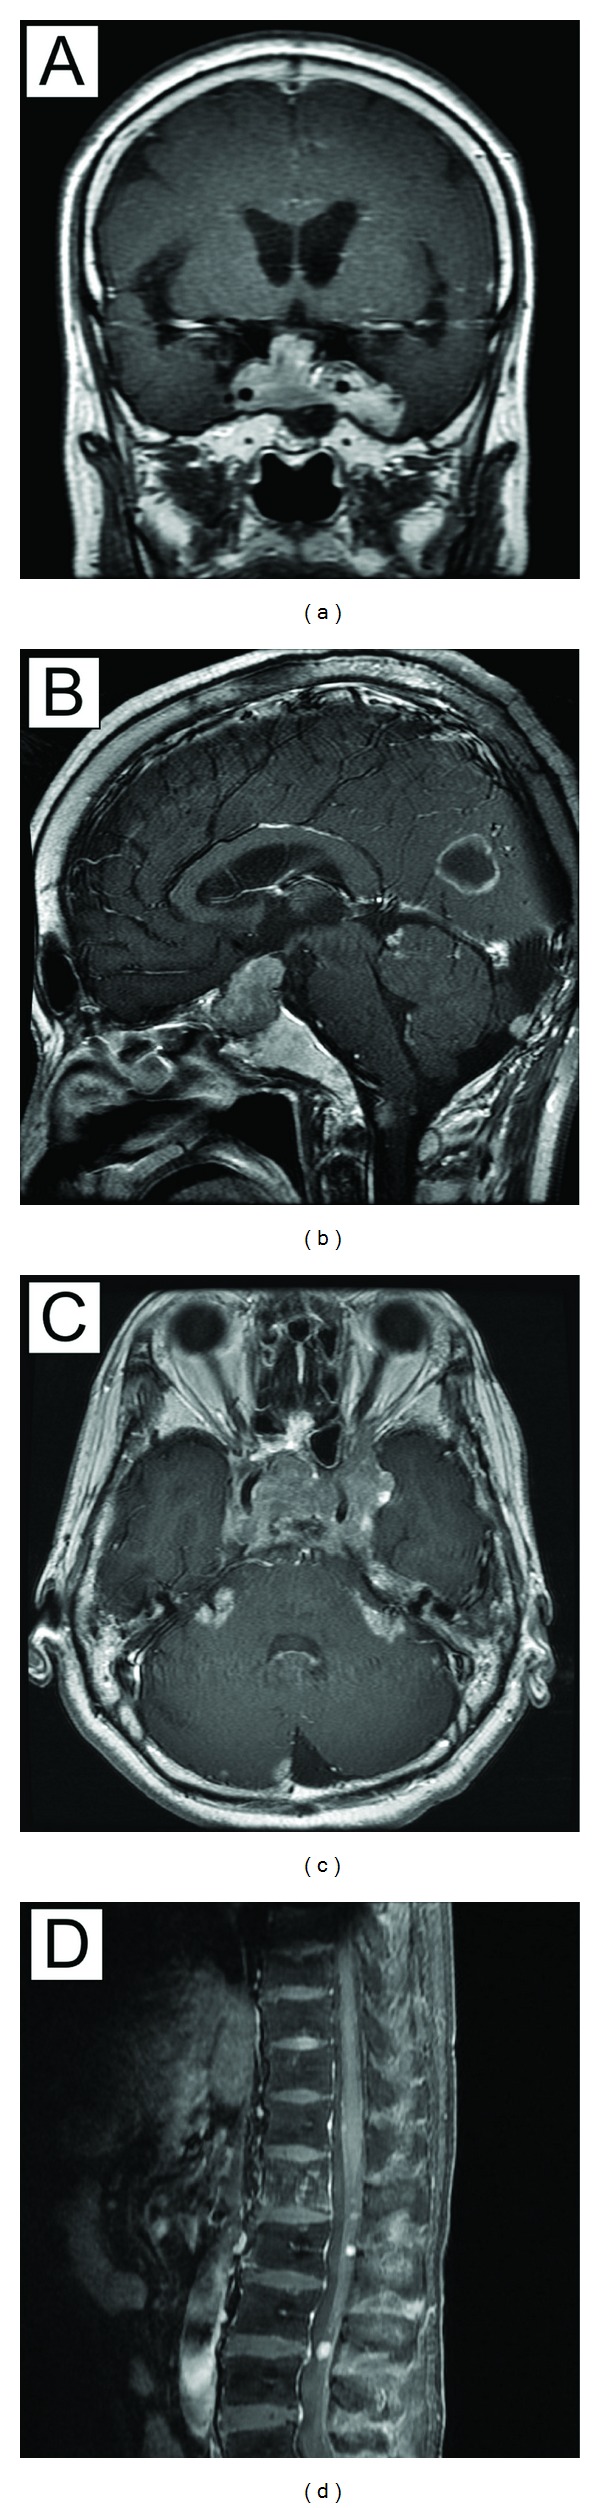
Pituitary carcinoma before the treatment. The pituitary tumor surrounds the bilateral cavernous sinus and protrudes close to the optic chiasm (detected by contrast-enhanced MRI). The tumor invades the left temporal lobe (a). Metastatic brain tumor in the occipital lobe shows ringlike enhancement (b). The pituitary tumor shows meningeal dissemination (c). Metastatic tumor in the L1 and diffuse meningeal dissemination (d).
